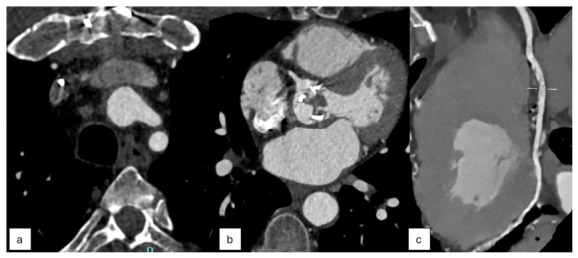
Examples of lesions detected by CCT: (a) paravalvular abscess surrounding the aortic root in a patient with prosthetic valve endocarditis, (b) a case of endocarditis involving a mechanical prosthetic aortic valve complicated by a pseudoaneurysm, (c) CTA showing an atherosclerotic plaque involving the right coronary artery 2.
